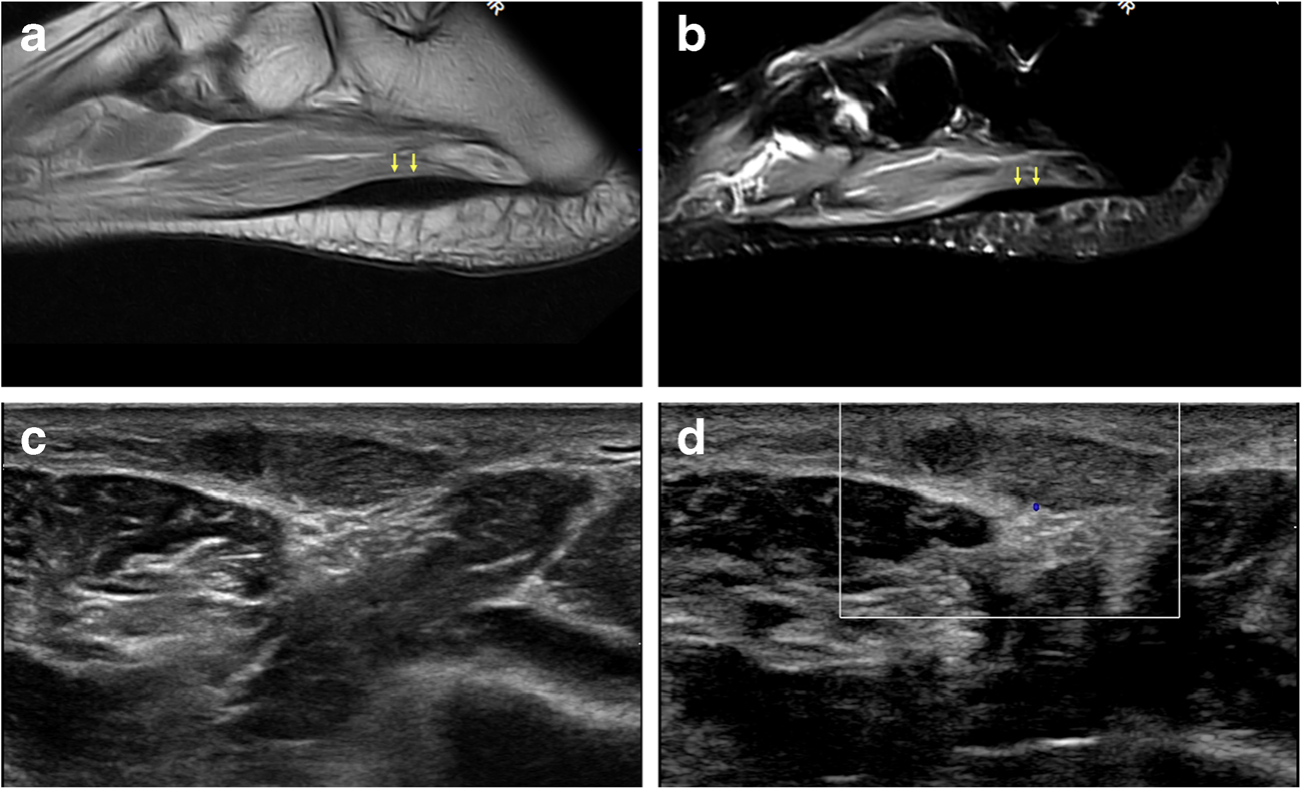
Ledderhose disease. MRI shows a fusiform thickening (*arrows*) in the distal portion of the PF with low signal intensity on both T1-weighted (a) and fluid-sensitive images (b). On ultrasound, a well-demarcated, hypoechoic nodule is demonstrated (c) with no increased internal vascularity (d)
